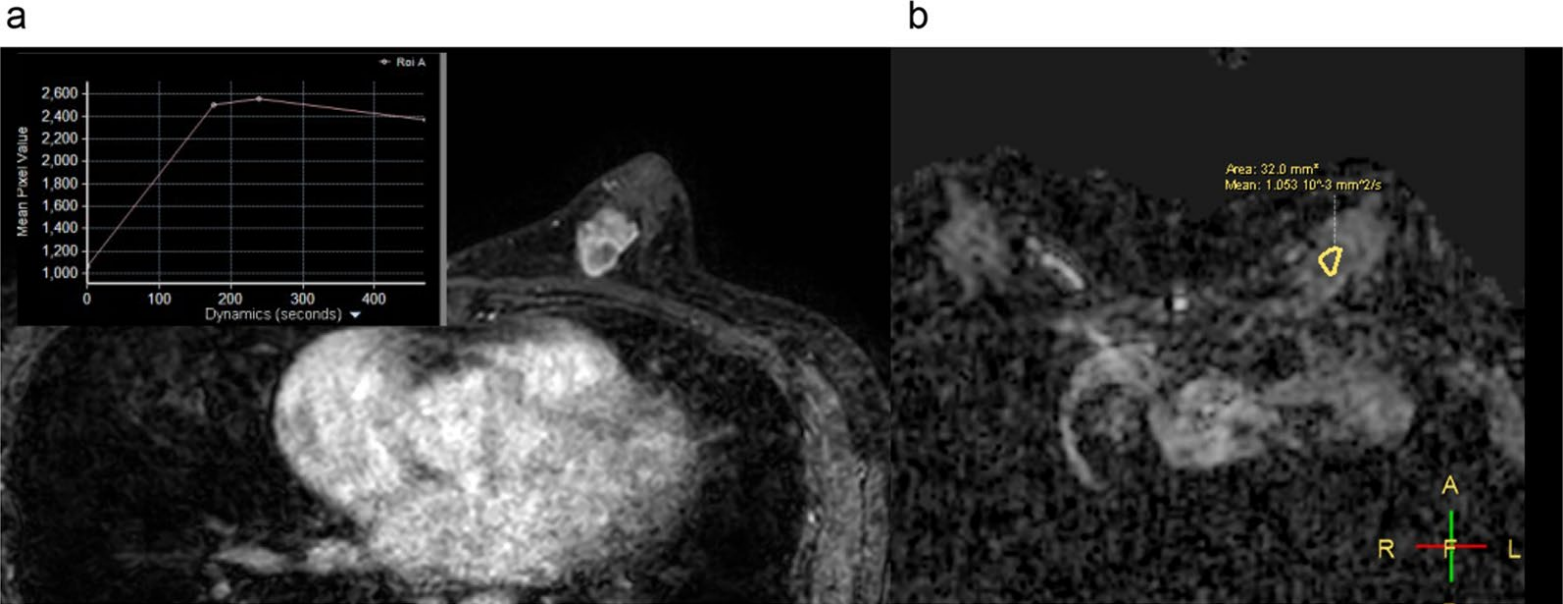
A MRI shows the irregular shaped mass enhanced with fast-washout kinetic pattern. B The tumor shows high signal of DWI with high diffusion restriction (ADC value 1.053 × 10^–3^ mm^2^/sec)
